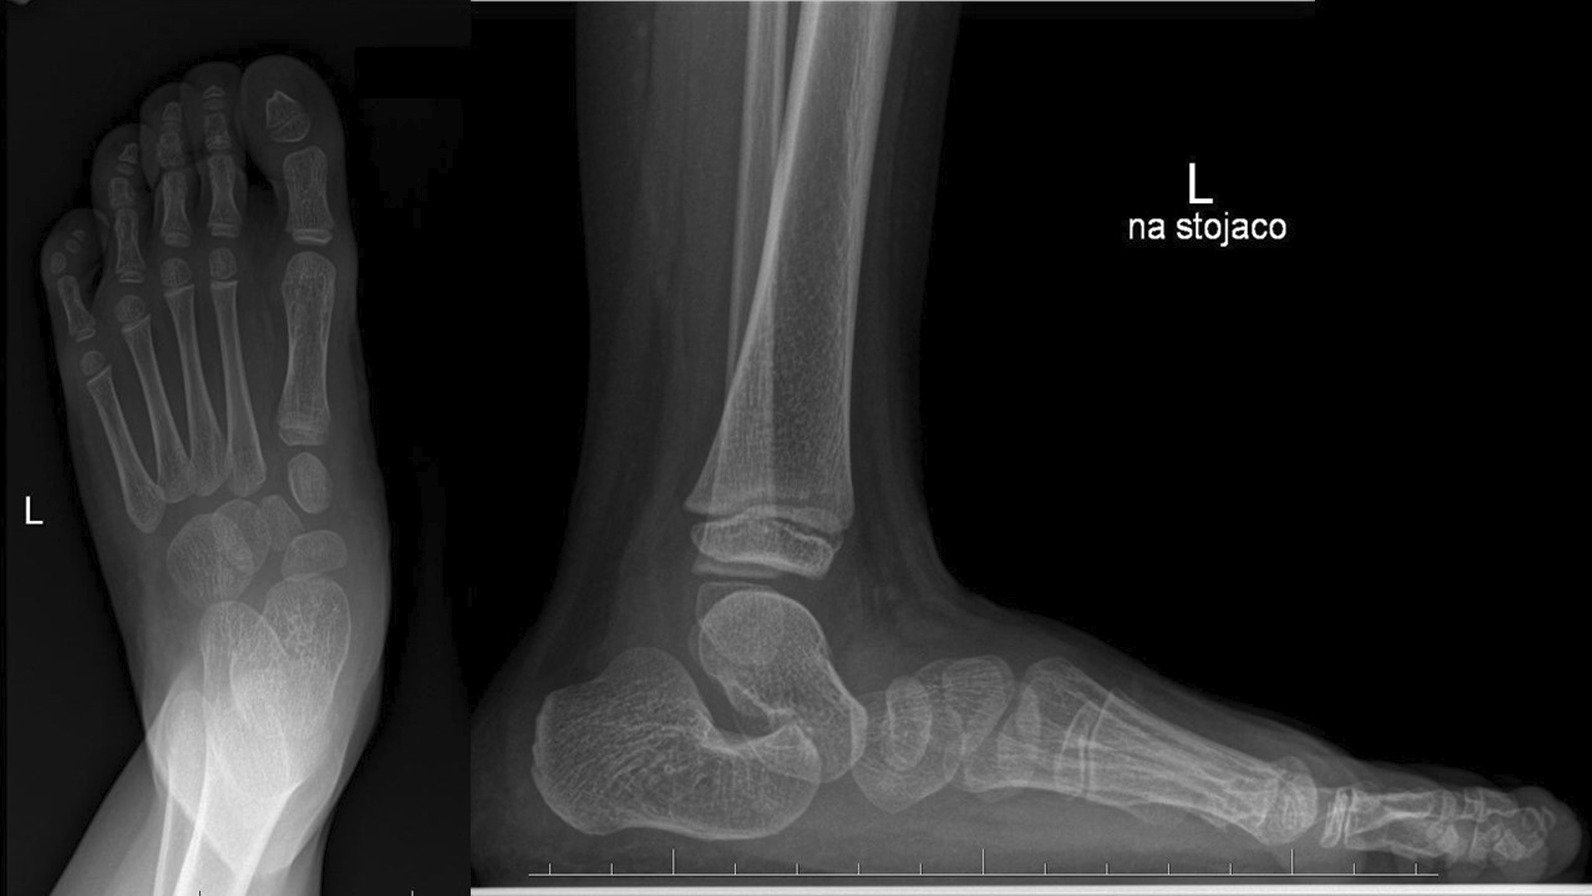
Case 1 AP and L X-ray at last follow-up. A recurrence of foot deformity is visible
